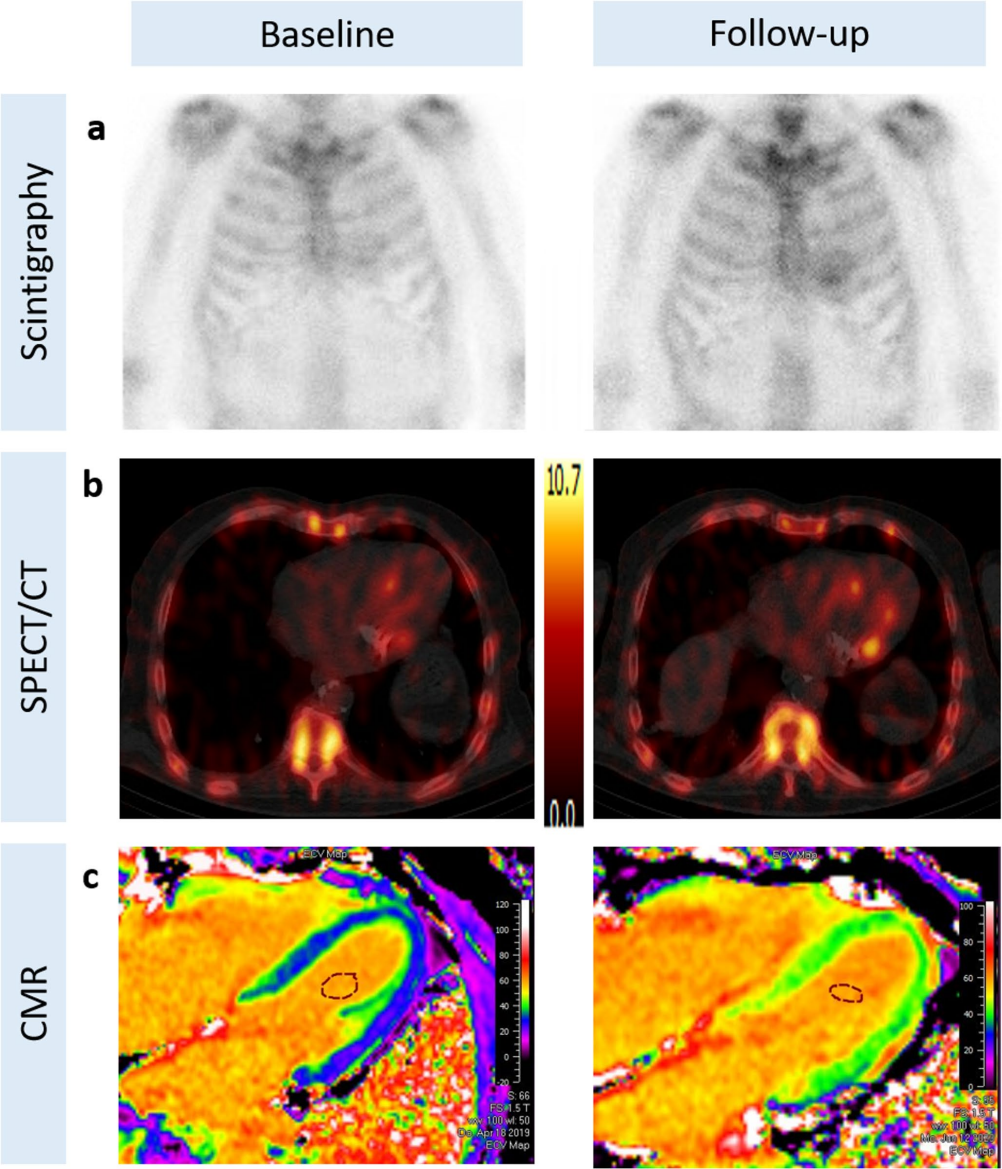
Representative nuclear medicine and CMR images showing the progression of ATTR-CM from baseline (left panels) to follow-up (right panels). Planar scintigraphic images (a) show the progression of the disease from Perugini grade 1 (baseline) to Perugini grade 2 (follow-up), confirmed also by SPECT/CT (b) and CMR ECV-maps (c). ATTR-CM, transthyretin amyloid cardiomyopathy; CMR, cardiac magnetic resonance; ECV, extracellular volume; SPECT/CT, single photon emission computed tomography/computed tomography
